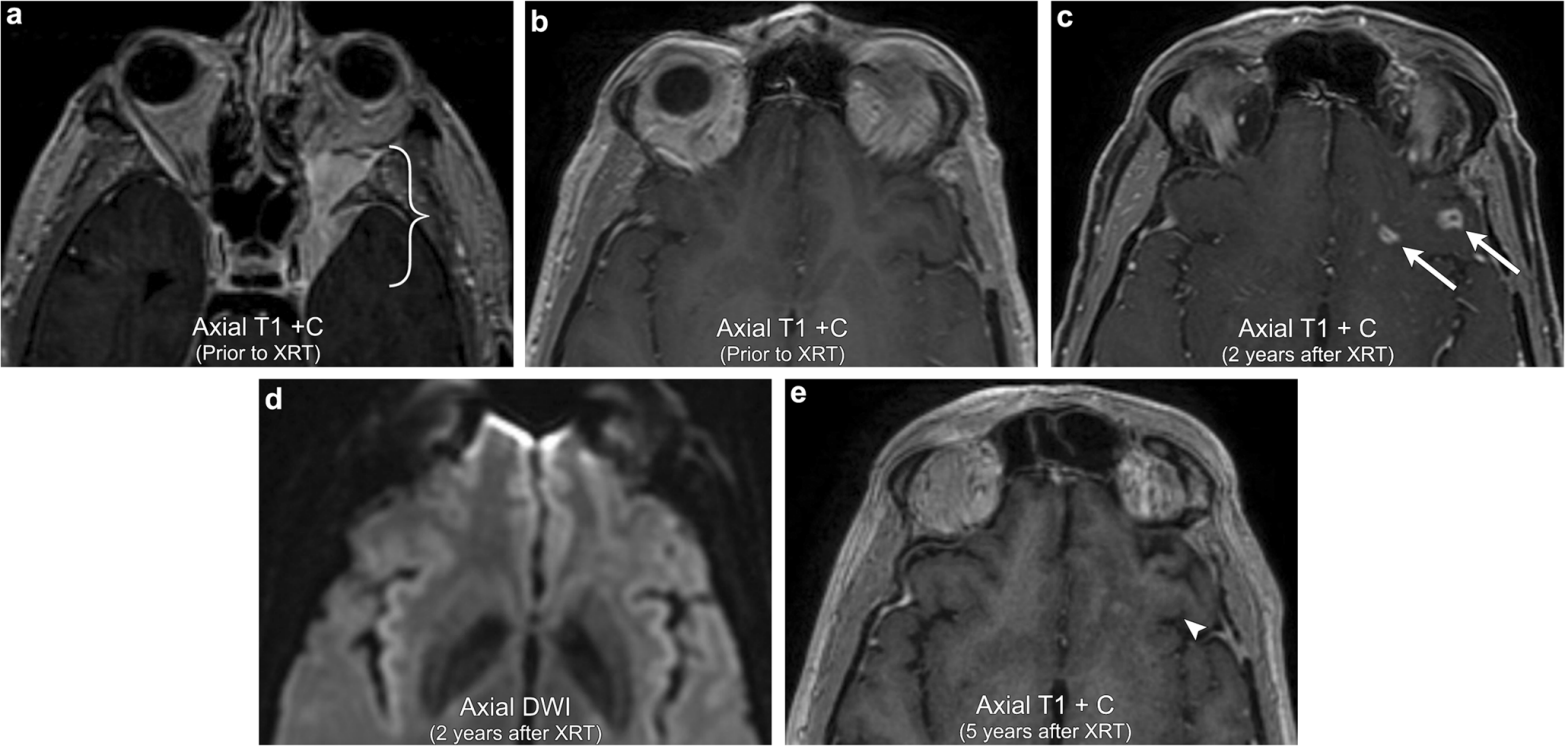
Radiation necrosis. A 58-year-old male with left sphenoid wing meningioma extending into the left cavernous sinus and orbital apex (bracket). The left basifrontal region at that time was unremarkable. Follow-up imaging 2 years after receiving radiation now demonstrates several irregular rim-enhancing lesions in the left basifrontal region (arrows). There is no abnormal corresponding DWI signal. Follow-up imaging 5 years after radiation now demonstrates resolution of the irregular enhancing lesions in the left basifrontal region (arrowhead), confirming radiation necrosis
